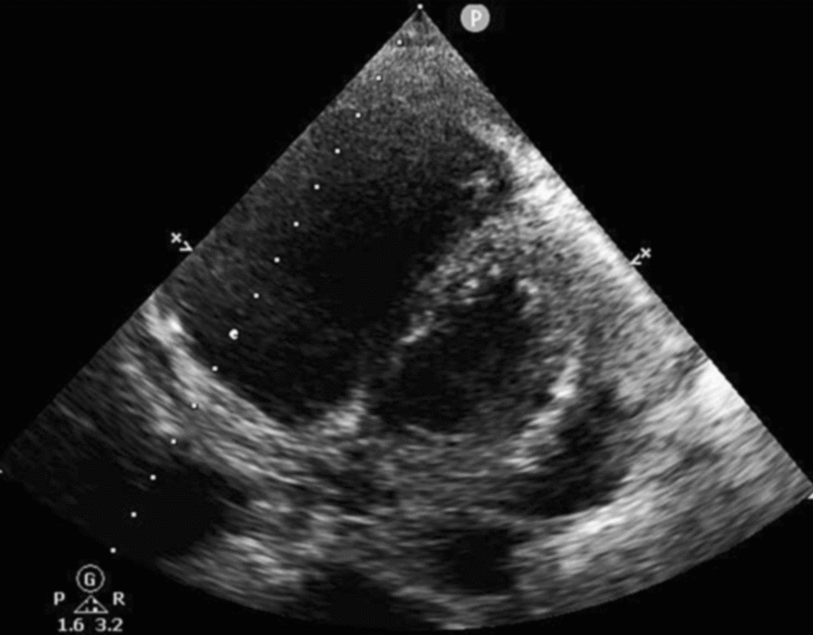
Short axis of left ventricular of transthoracic echocardiography
